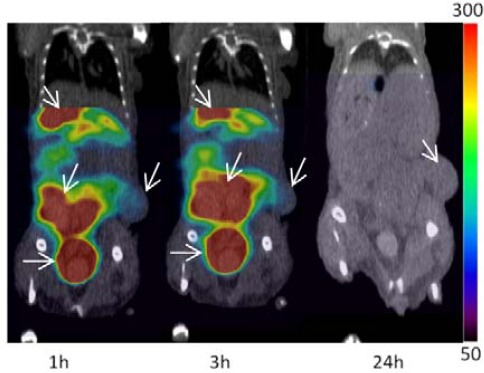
SPECT/CT image of [^125^I]PYK in U87MG.ΔEGFR xenograft at 1 hr, 3 hr, 24 hr post injection. The colon, liver, bladder and tumor were indicated by arrows. The thyroid was out of field of view in these SPECT images.
